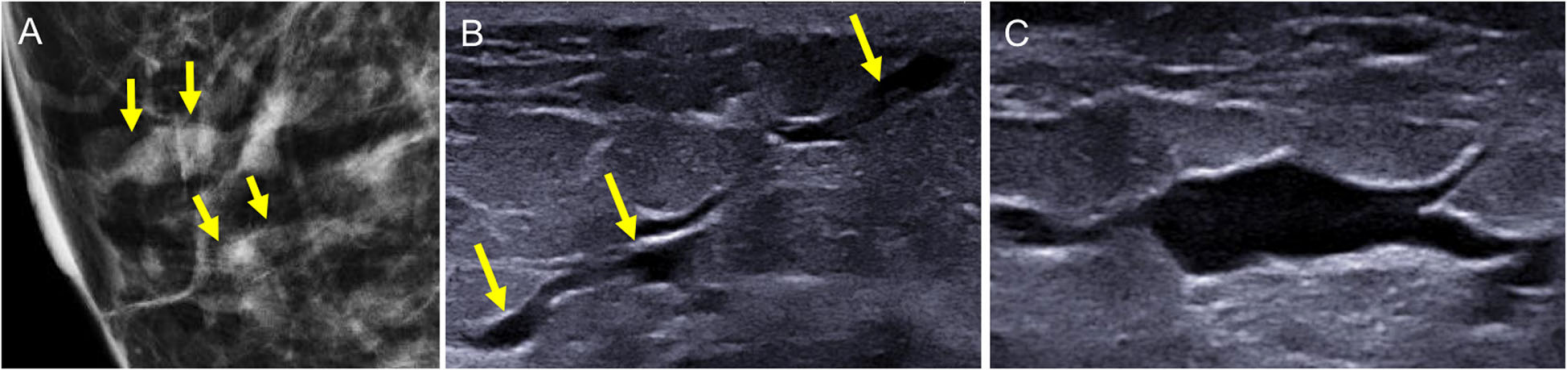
Ductal ectasia. Different examples of ductal ectasia without interior contents seen on mammography (a) and ultrasound (b, c)
